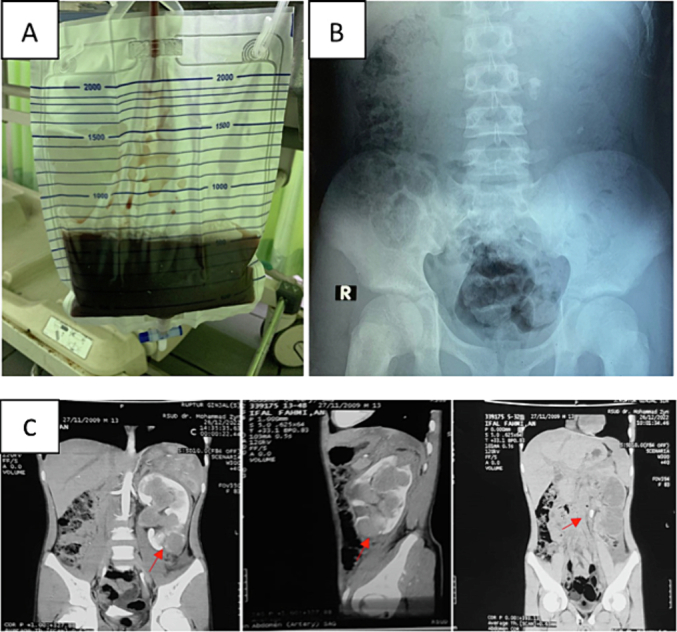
(A) Urine output was 250 cc/12 h with gross hematuria; (B) On abdominal X-ray examination was found multiple radiopaque shadows with the size of 1.4 × 0.7 cm at the VL 3–4 level on the left side; (C) Abdominal CT scan with intravenous pyelogram (IVP) revealed severe hydronephrosis with hydroureter and multiple stones in the left lower calyx pole measuring 0.6 cm (880 HU), as well as stones in the ureteropelvic junction (UPJ) (1182 HU).
